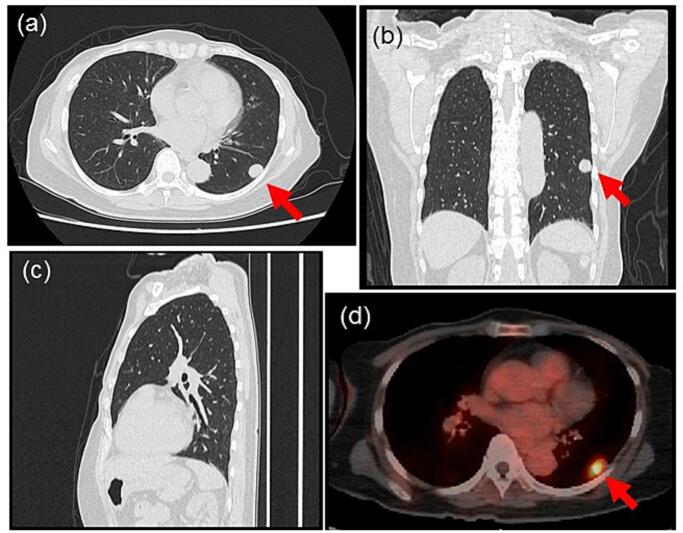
Preoperative chest computed tomography (CT) and FDG-PET/CT images. (a) CT, axial section image; (b) CT, coronal section image: primary lung adenocarcinoma in the left lower lobe S^9^a, 18 × 12 × 16 mm (red arrow); (c) CT, sagittal section image showing a poorly developed interlobar fissure of the left lung. (d) FDG-PET/CT: FDG uptake into the cancer is significant (SUVmax: 7.44) (red arrow). (For interpretation of the references to colour in this figure legend, the reader is referred to the web version of this article.)
